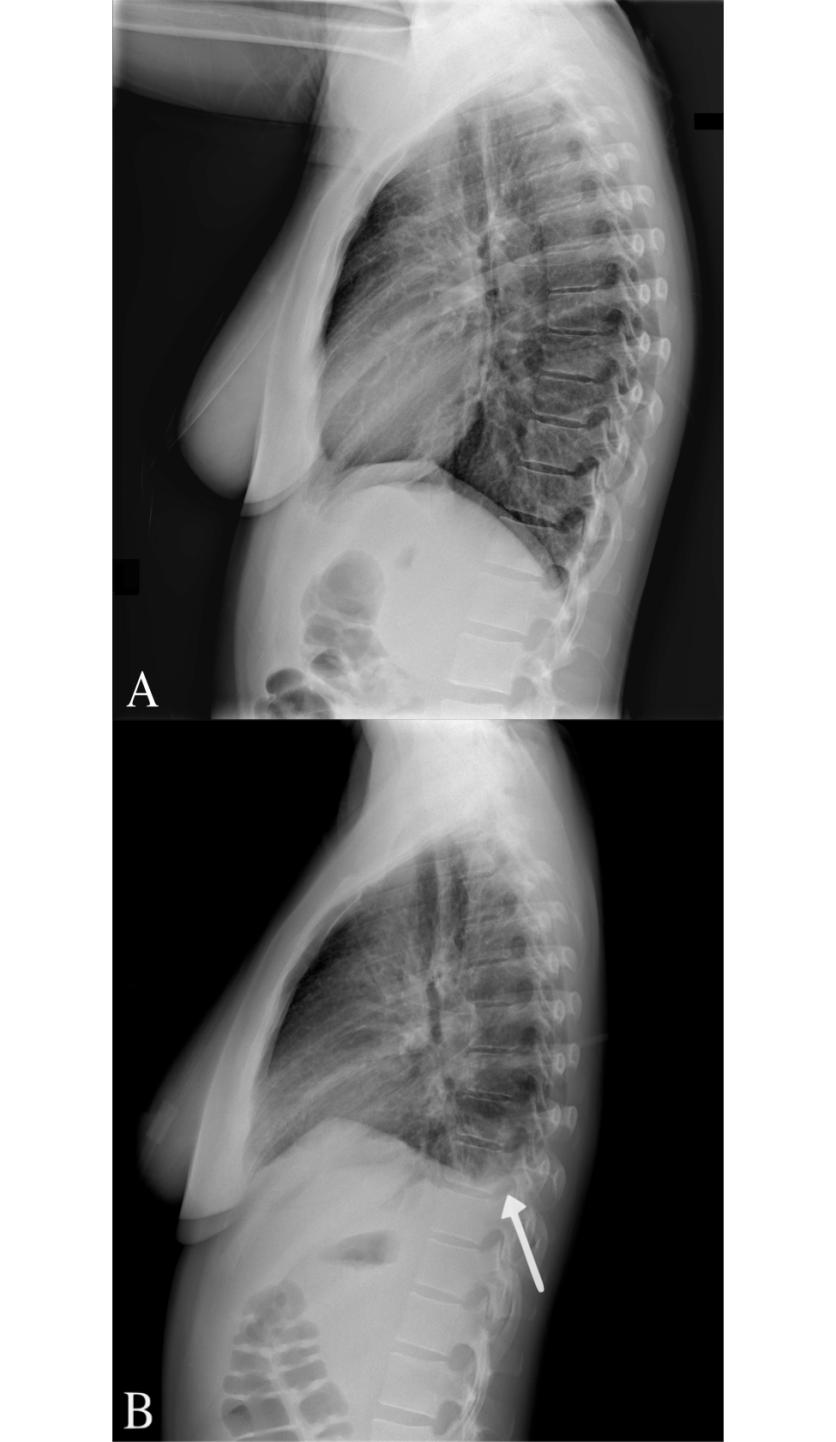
Radiography shows development of an infiltrate. (A) Lateral chest radiography at admission to the hospital shows no abnormalities. (B) Three days later, radiography reveals a left-sided infiltrate (white arrow).
